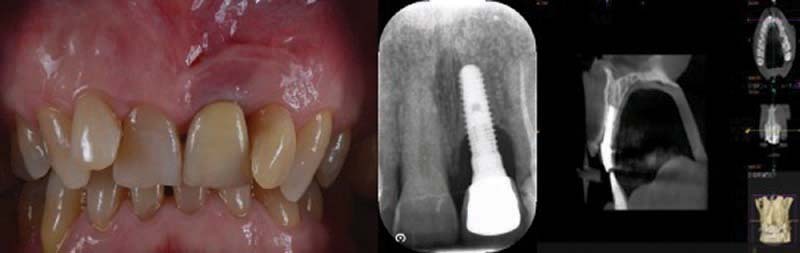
Clinical and radiographic image (2D, 3D) at baseline. 2D, two-dimensional; 3D, three-dimensional.
